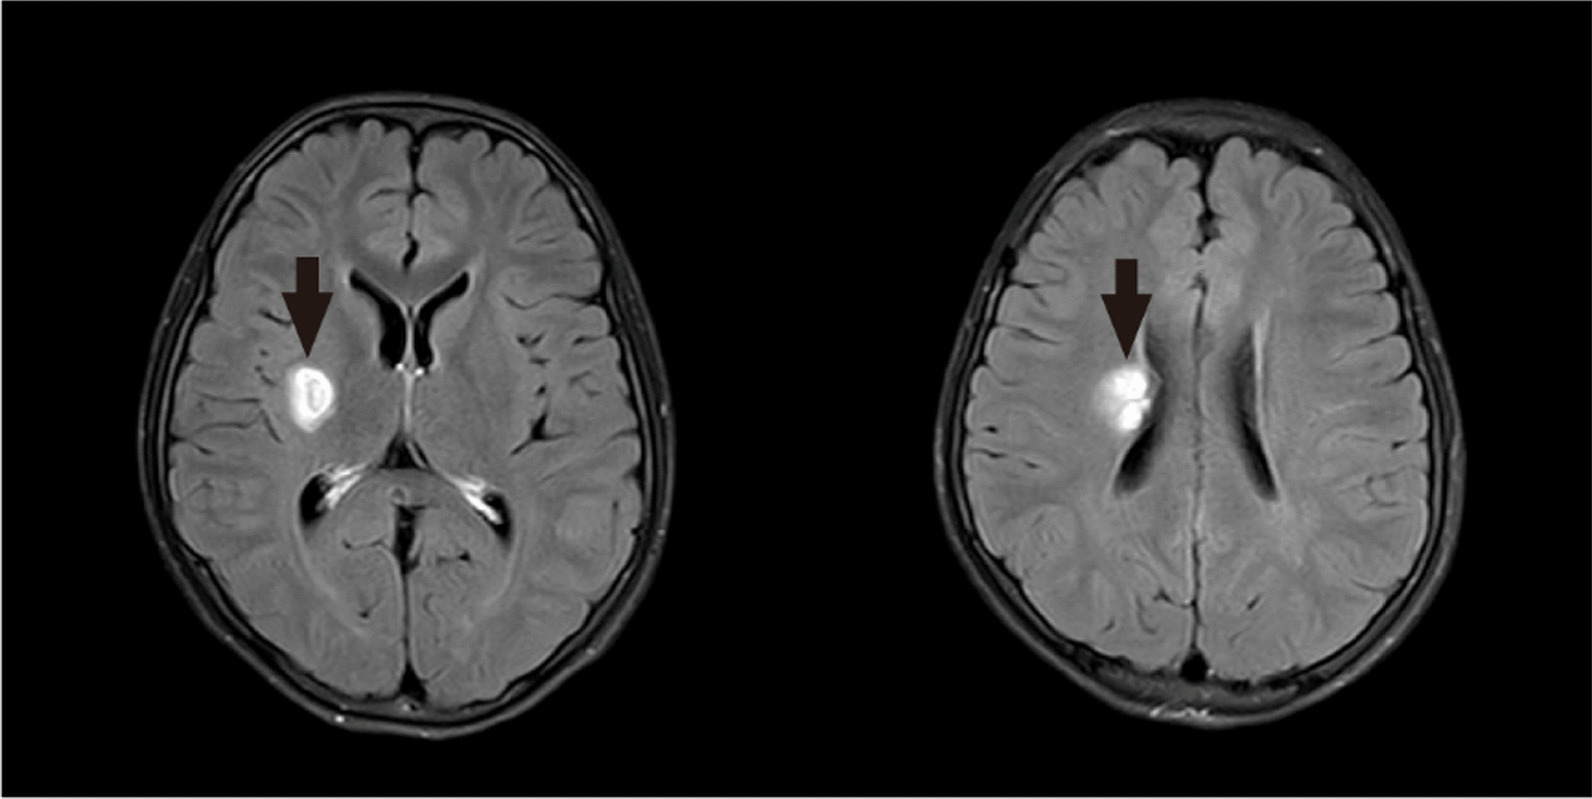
Cranial magnetic resonance images obtained from the patient. The black arrow shows the right side of the basal ganglia region-lateral ventricle lesion
